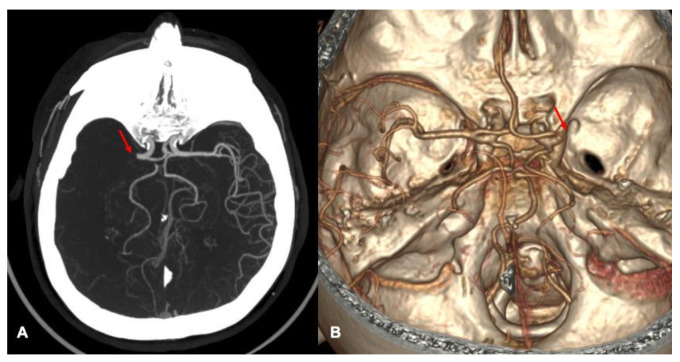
Patient case example: Evaluation of multimodal CT imaging. A 73-year-old female patient’s CTA imaging revealed an occlusion in the proximal segment of the right MCA (M1 segment), with malignant collaterals (0 points). (A) Maximum intensity projection (MIP) image showing the occlusion of the M1 segment of the right MCA, indicated by an arrow. (B) 3D CTA reconstruction highlighting the M1 segment occlusion (indicated by an arrow), providing enhanced visualisation of the location and extent of the thrombus.
